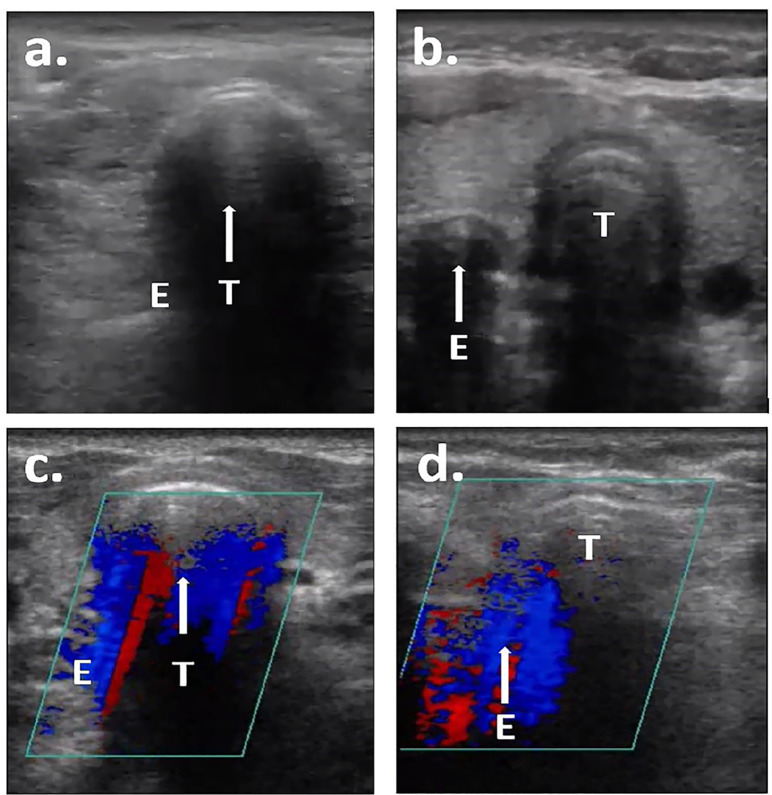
Representative screenshots from online assessment videos: a) tracheal intubation in grayscale (single air-mucosal interface with reverberation artifact within the trachea); b) esophageal intubation in grayscale (double air-mucosal interface); c) tracheal intubation in color Doppler imaging (CDI) (color signal deep to the trachea); and d) esophageal intubation in CDI (color signal deep to the esophagus). Arrow indicates location of intubation. *T*, trachea; *E*, esophagus.
